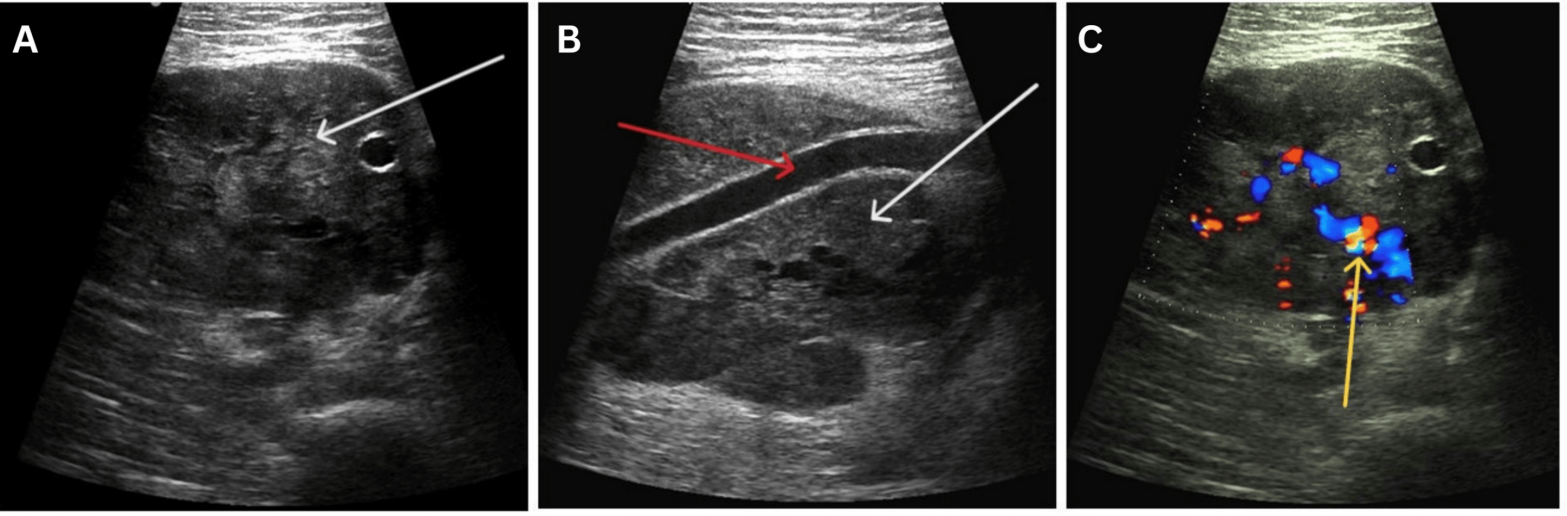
Ultrasonography (USG) of the swelling at the anteromedial compartment of the thigh revealing a soft tissue lesion (white arrow) encasing the superficial femoral artery (red arrow). A) Transverse view; B) Longitudinal view; C) Doppler USG of the same lesion shows increased vascularity (yellow arrow).
